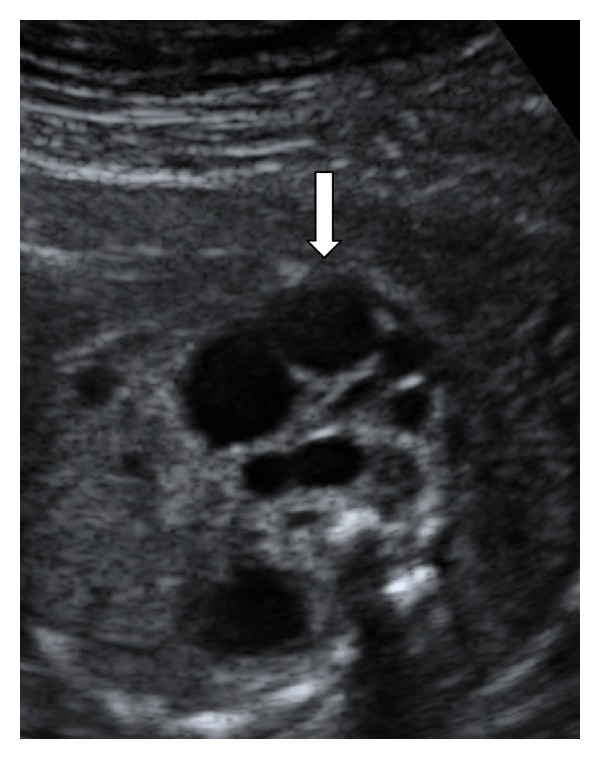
Transabdominal ultrasound showing a dysplastic (multicystic) kidney (arrow).
